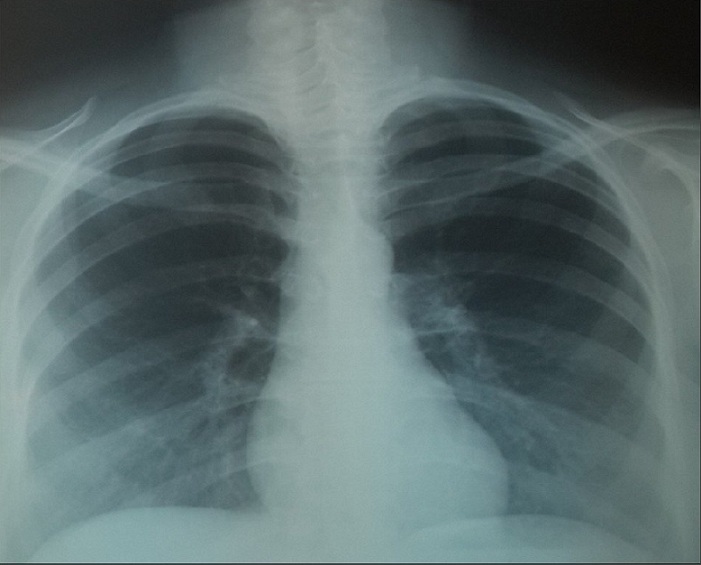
Radiographie thoracique sensiblement normale (après traitement)
